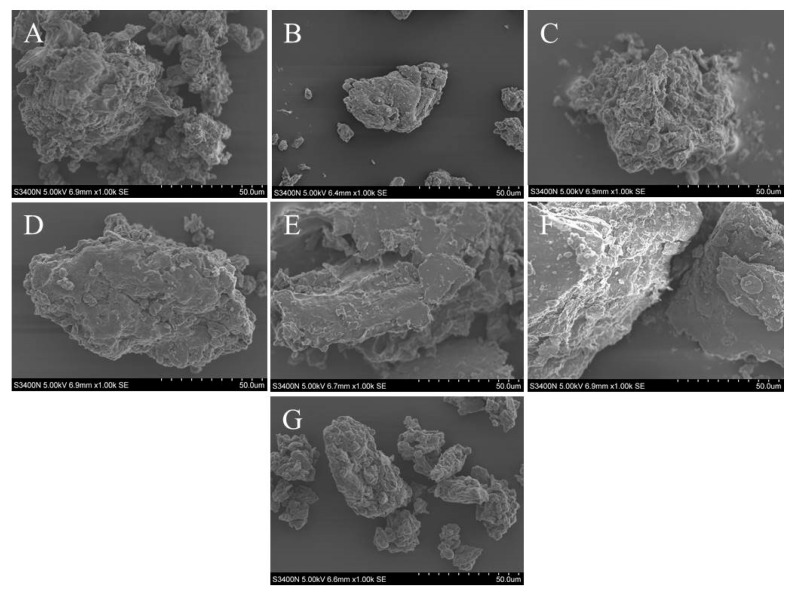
The powder after different methods extraction exhibited various degrees of fragmentation with 1.00k SEM: (A) ultrasound-assisted DES-6 extraction; (B) decoction piece; (C) ultrasonic water extraction; (D) distilled water extraction; (E) hot water reflux; (F) thermal extraction; (G) the untreated raw medicinal powder.
